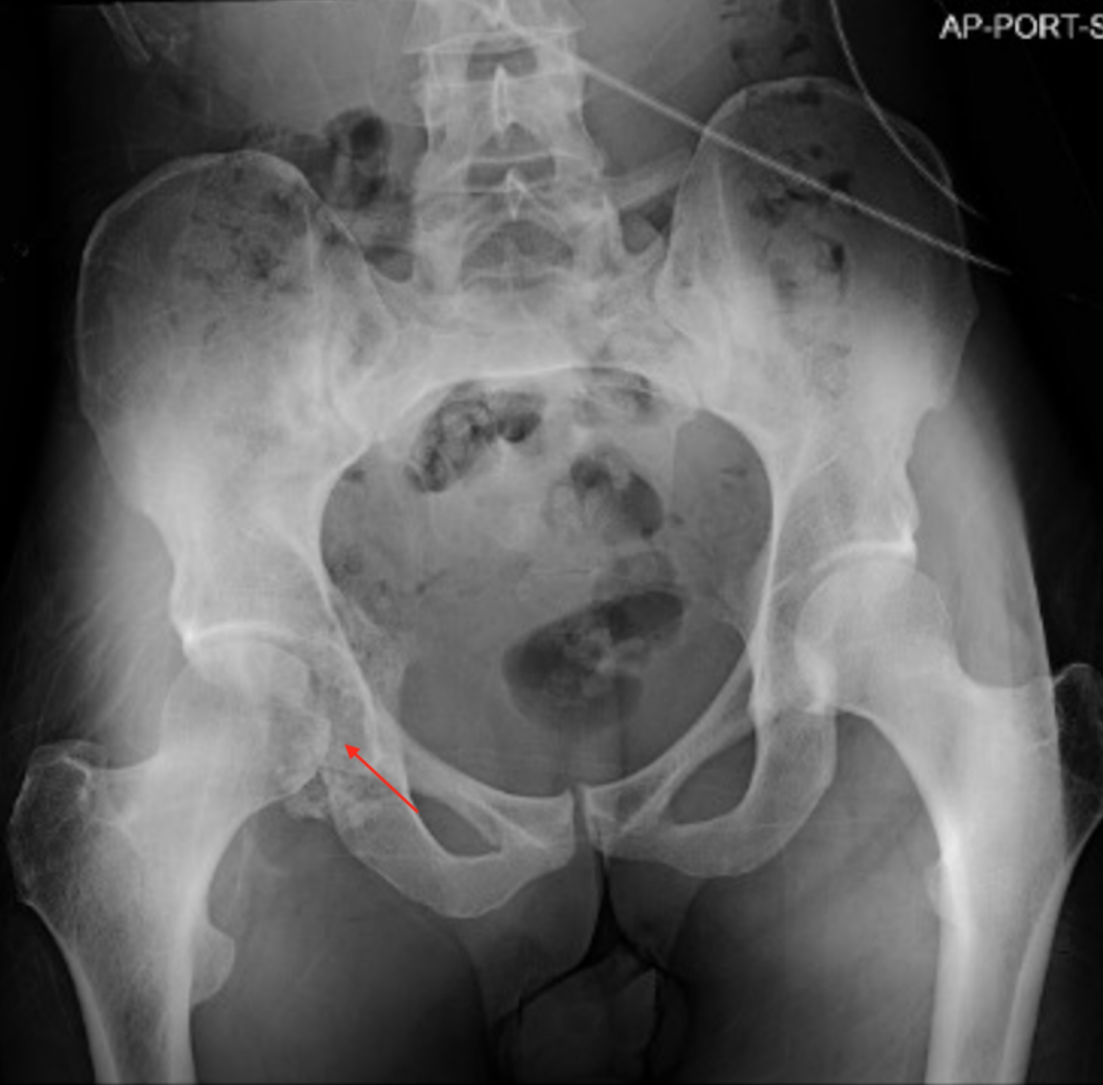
Anterior-posterior X-ray of the pelvis demonstrating lucency and bone destruction associated with the right acetabulum, suspicious for metastatic disease
